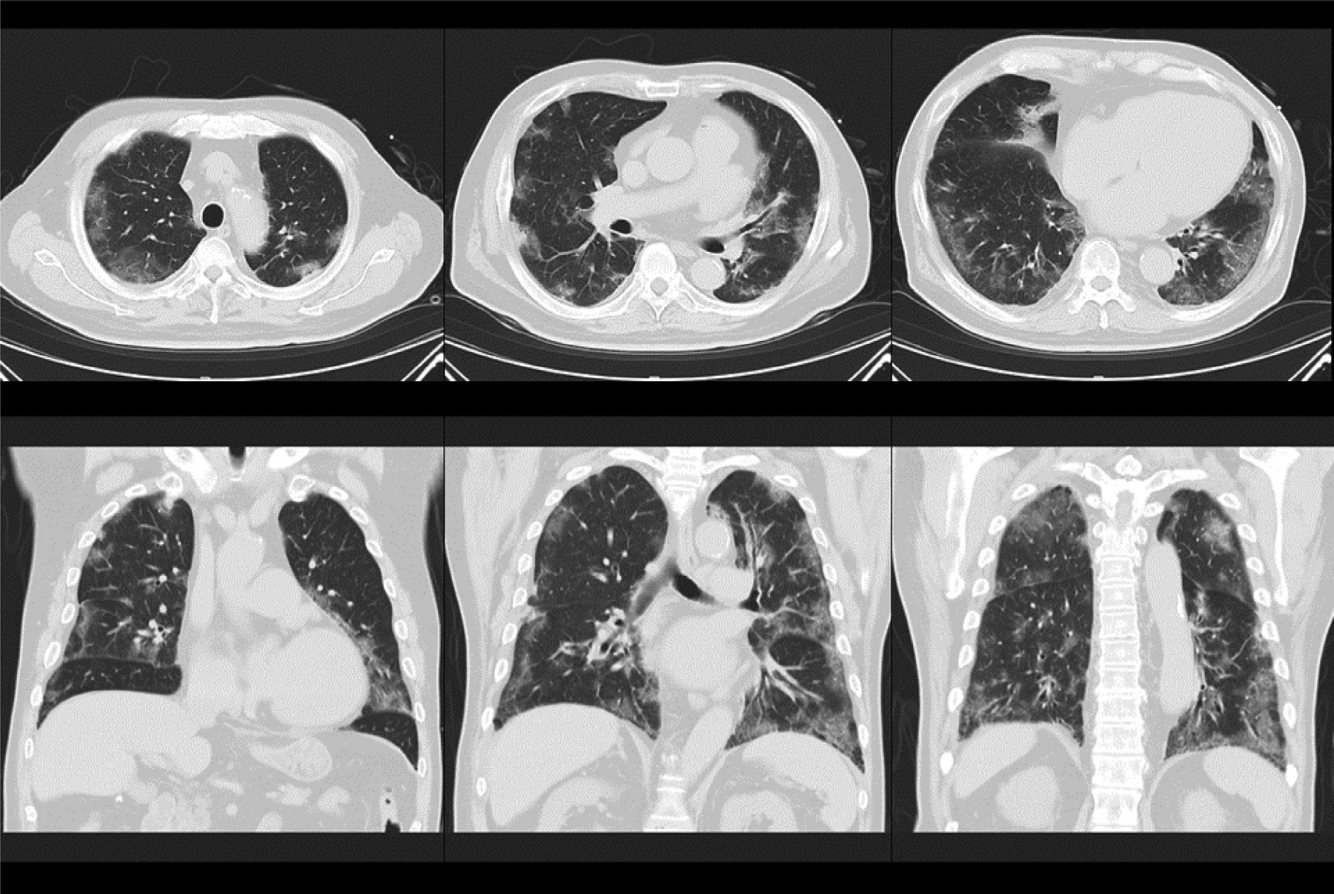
Chest computed tomography (CT) showing bilateral consolidating and infiltrative, peripheral ground-glass opacities, characteristic of coronavirus disease 2019 (COVID-19).
